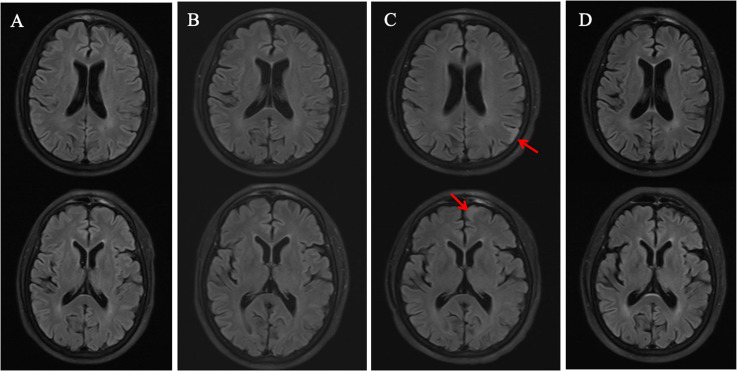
MRI changes at different admission. (A) First visit: No significant abnormalities were seen on the brain MRI. (B) Second visit: No significant abnormalities were seen on the brain MRI. (C) Third visit: T2-hyperintense signal (arrows) in the left frontal and parietal lobes. (D) Follow-up after treatment: The previously observed T-2 hyperintensities in the left frontal and parietal lobes had resolved.
